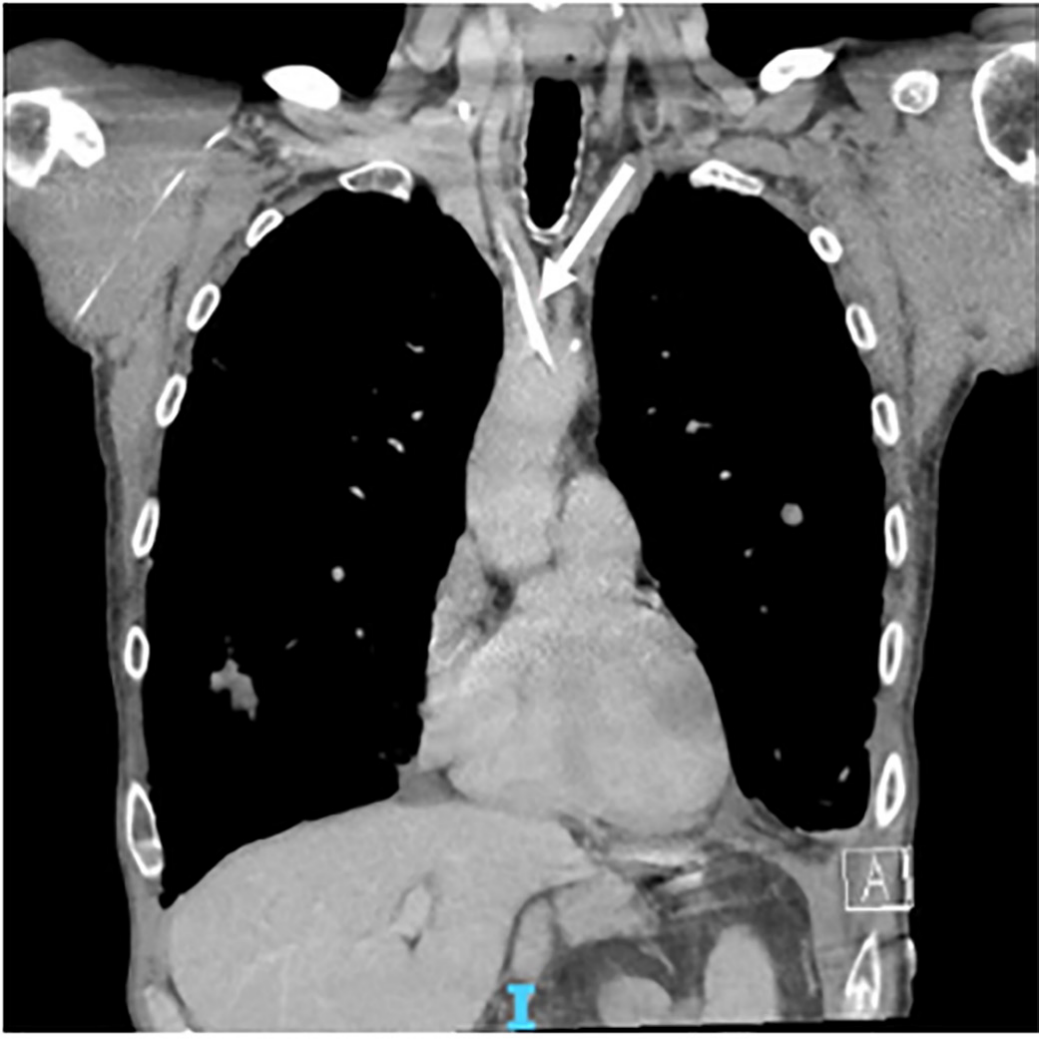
Coronal view of a computed tomography angiogram depicting the central venous
